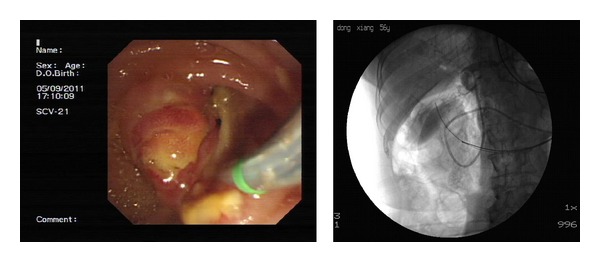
Pre-ampullary perforation by cutting. The kidney shadow was shown by X-ray.
